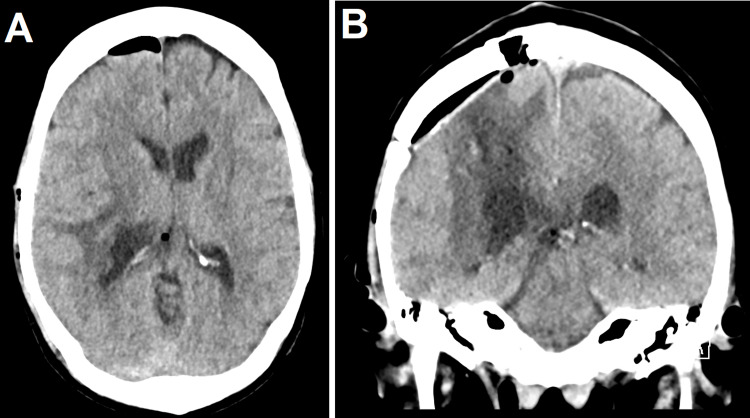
Postoperative axial (A) and coronal (B) slices of computed tomography scan of the head demonstrating expected post-surgical changes.
